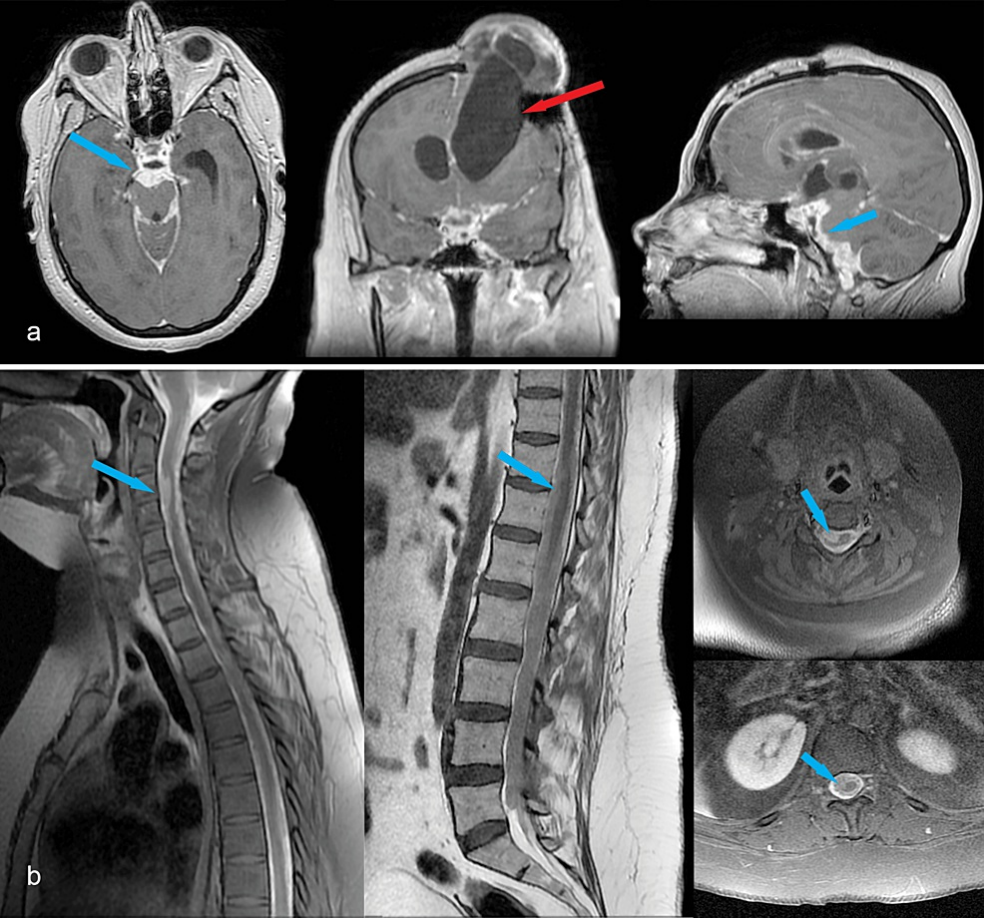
a: axial, coronal, and sagittal contrast-enhanced T1 MRI show the right ventricle collapsed, the left ventricle dilated and deformed (red arrow); basal and infratentorial cisterns, cranial nerves, and both sylvian fissures present pseudo-nodular, contrast-enhancing thickenings (blue arrows); b: sagittal and axial spinal T2 MRI depicts similar lesions throughout the entire spinal dura mater (blue arrows)
